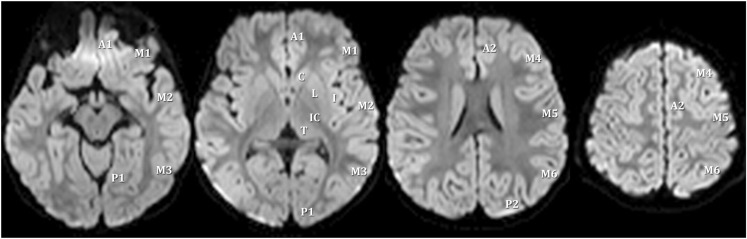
Scoring areas for the modified pediatric ASPECTS. A1, proximal anterior cerebral artery territory; P1, inferior portion of posterior cerebral artery territory; M, middle cerebral artery; C, caudate; L, lentiform; I, insula; IC, internal capsule; T, thalamus; P2, superior portion of posterior cerebral artery territory; A2, distal anterior cerebral artery territory.
